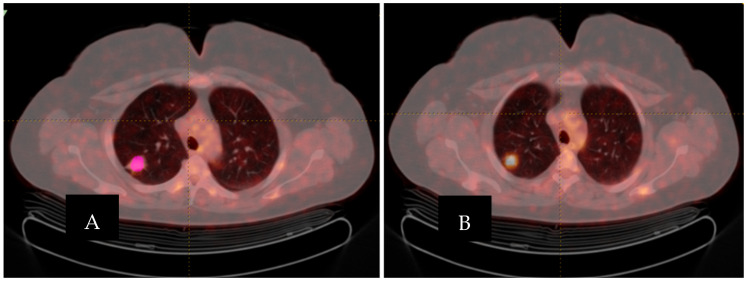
(A). PET/CT fusion image, (B). Segmentation (with pink color) of the lung lesion in the ^18^F-FDG PET/CT with LIFEx software.
